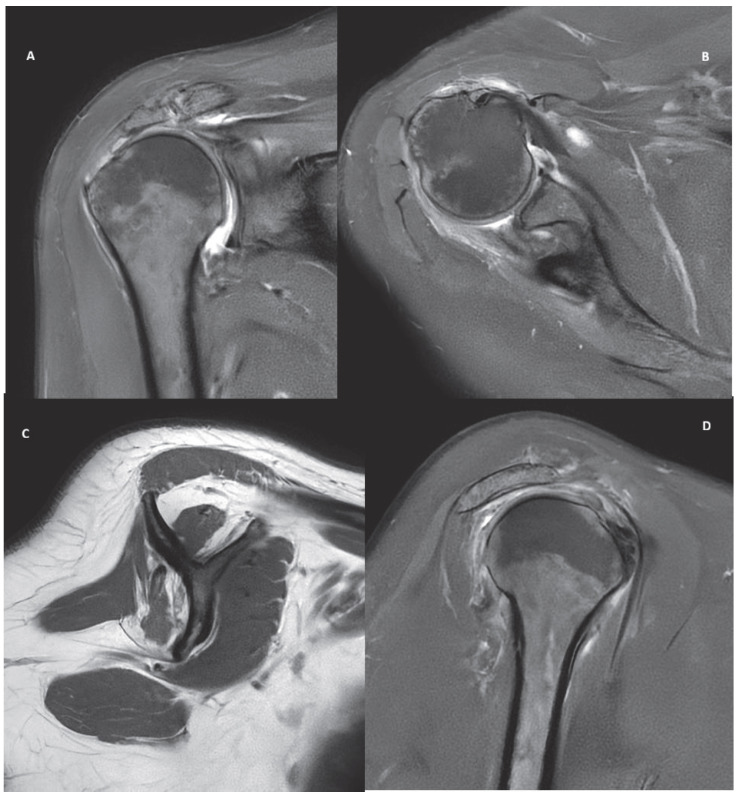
MRI images of an irreparable posterosuperior rotator cuff tear with supraspinatus, infraspinatus involvement and Fuchs > 3 fat atrophy in coronal (A), axial (B) and sagittal (C,D) view.
